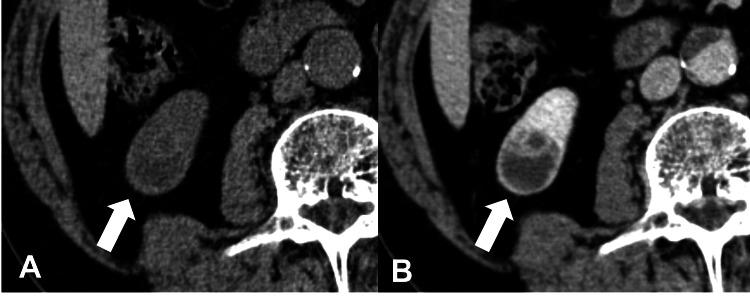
CT images of a 68-year-old man with clear cell RCC A: unenhanced image; B: enhanced image in nephrographic phase On axial UE-CT, a 2.4-cm heterogeneous hypodense mass with a thick wall and a mural nodule is seen in the lower pole of the right kidney (arrow). The enhanced image demonstrates a well-enhancing mural nodule within the mass, suggestive of Bosniak category IV. Pathologic examination confirmed that the mass was a clear cell RCC RCC: renal cell carcinoma; UE-CT: unenhanced computed tomography
